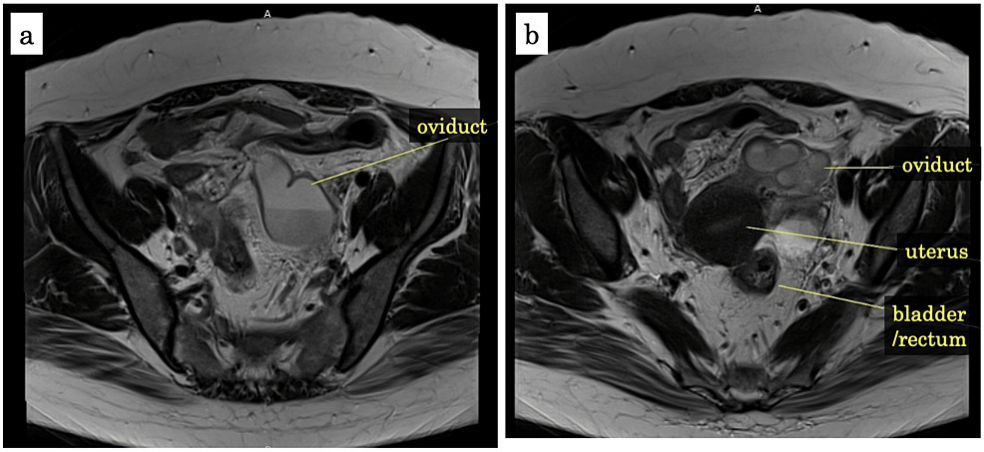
MRI on day 4 after admission MRI demonstrated that the fluid level was inside the left oviduct, and the left oviduct was enlarged like consecutive cysts.
